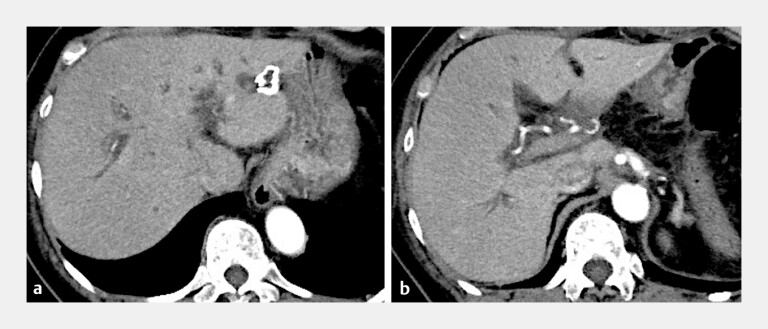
Arterial phase of contrast-enhanced computed tomography.
